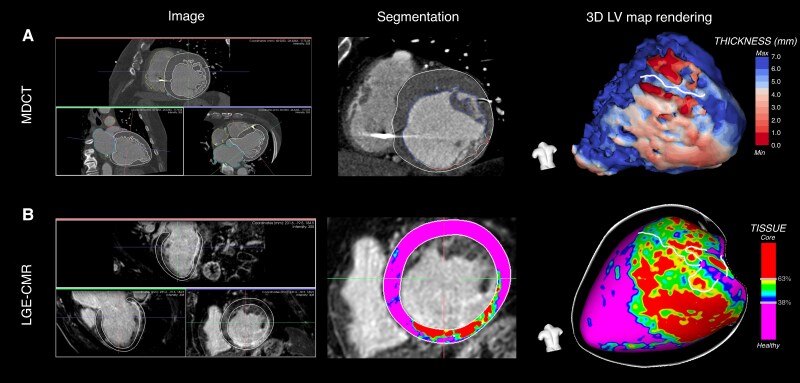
Cardiac magnetic resonance- and MDCT-derived data post-processing and NSC maps reconstruction of a post-myocardial infarction patient. (*A*) Pre-procedural MDCT-derived image data post-processing and 3D LV wall thinning map rendering with computed tomography channel detection in a patient with ischaemic cardiomyopathy who experienced an inferior myocardial infarction. (*B*) Pre-procedural CMR-derived image data post-processing and pixel signal intensity map rendering with BZCs detection in the same patient. LGE-CMR, late gadolinium enhancement-cardiac magnetic resonance; LV, left ventricle; MDCT, multi-detector computed tomography.
